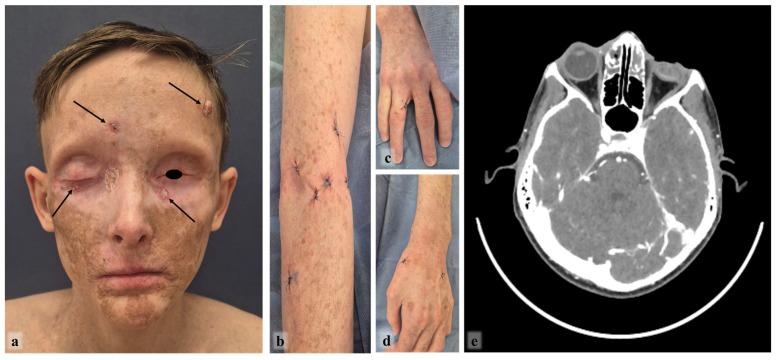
Examination of the patient (February 2026, 17 y.o.): growth of residual tumor and new foci of SCC (indicated by arrows) of the facial skin (a); surgically removed foci of SCC of the skin in the upper limbs (b–d); computed tomography—additional tissues in the frontal area and uneven accumulation of contrast (e).
